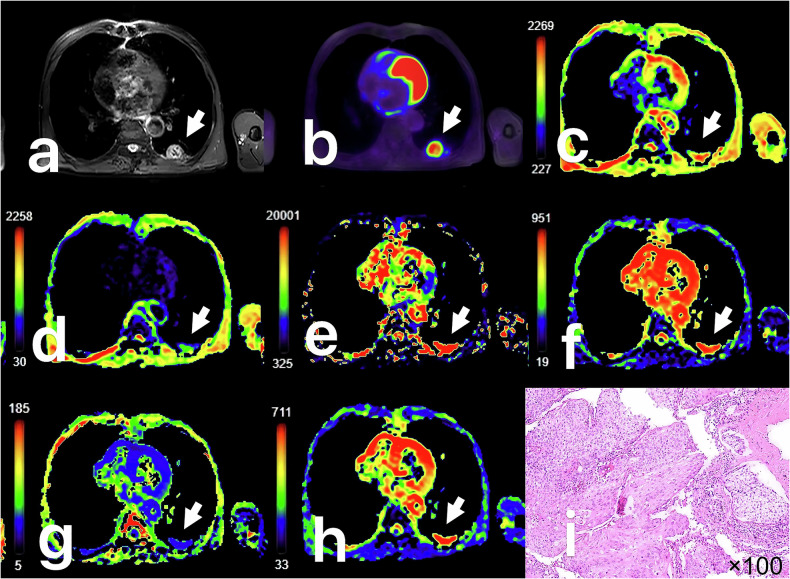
Male, 66 years old, with squamous carcinoma (SCC) in the left upper lobe, size 2.6 × 2.2 × 2.4 cm. a Image of axial T2WI with fat saturation pre-pulse. b Fusion image of the SUV map and the ultrashort echo time map. c Pseudo colored map of ADCstand. d Pseudo colored map of D. e Pseudo colored map of D*. f Pseudo colored map of f. g Pseudo colored map of MK. h Pseudo colored map of MD. i Pathological image that displays positive lymphovascular invasion
